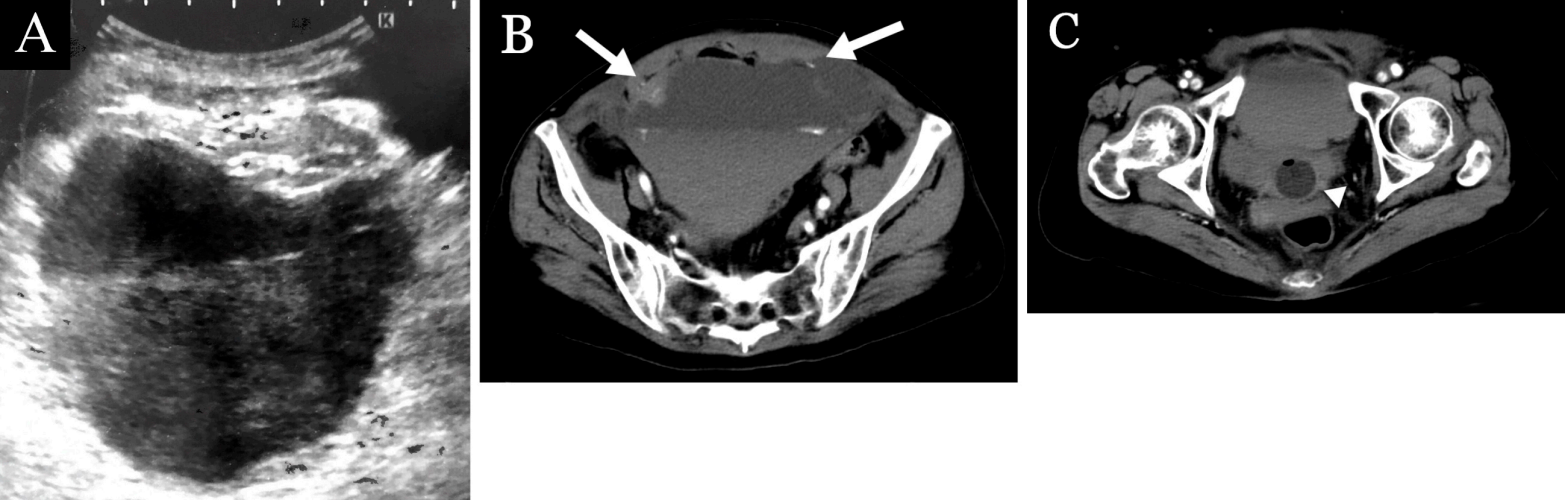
a. An ultrasound image resembling urinary bladder hematoma. b. Dynamic contrast-enhanced computed tomography (DCE-CT) showing a large hematoma in the rectus sheath, which extended below the arcuate line, with active bleeding and the hematocrit effect. There was evidence of contrast agent leakage between the rectus abdominis muscle and the hematoma. (arrows) c. A DCE-CT showing the hematoma compressing the urinary bladder, which contains a Foley catheter (arrowhead) (“pseudobladder sign”).
